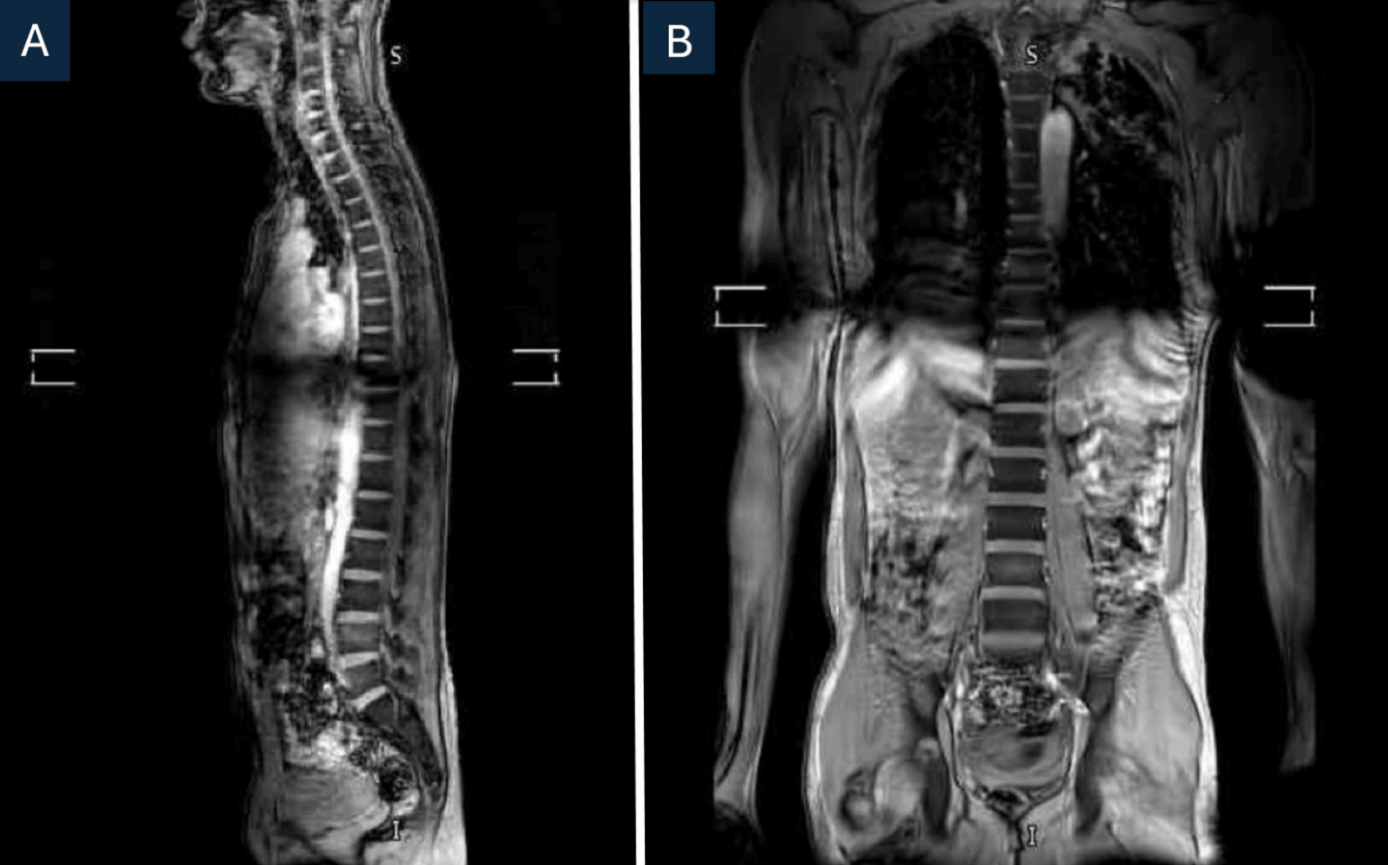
MRI thoracolumbar spine with contrast showing no evidence of spinal infection or cord involvement. (A) Sagittal T1-weighted image demonstrating normal vertebral alignment and spinal cord signal. (B) Coronal T1-weighted image showing intact vertebral bodies and paraspinal soft tissues without abscess or inflammatory changes.
